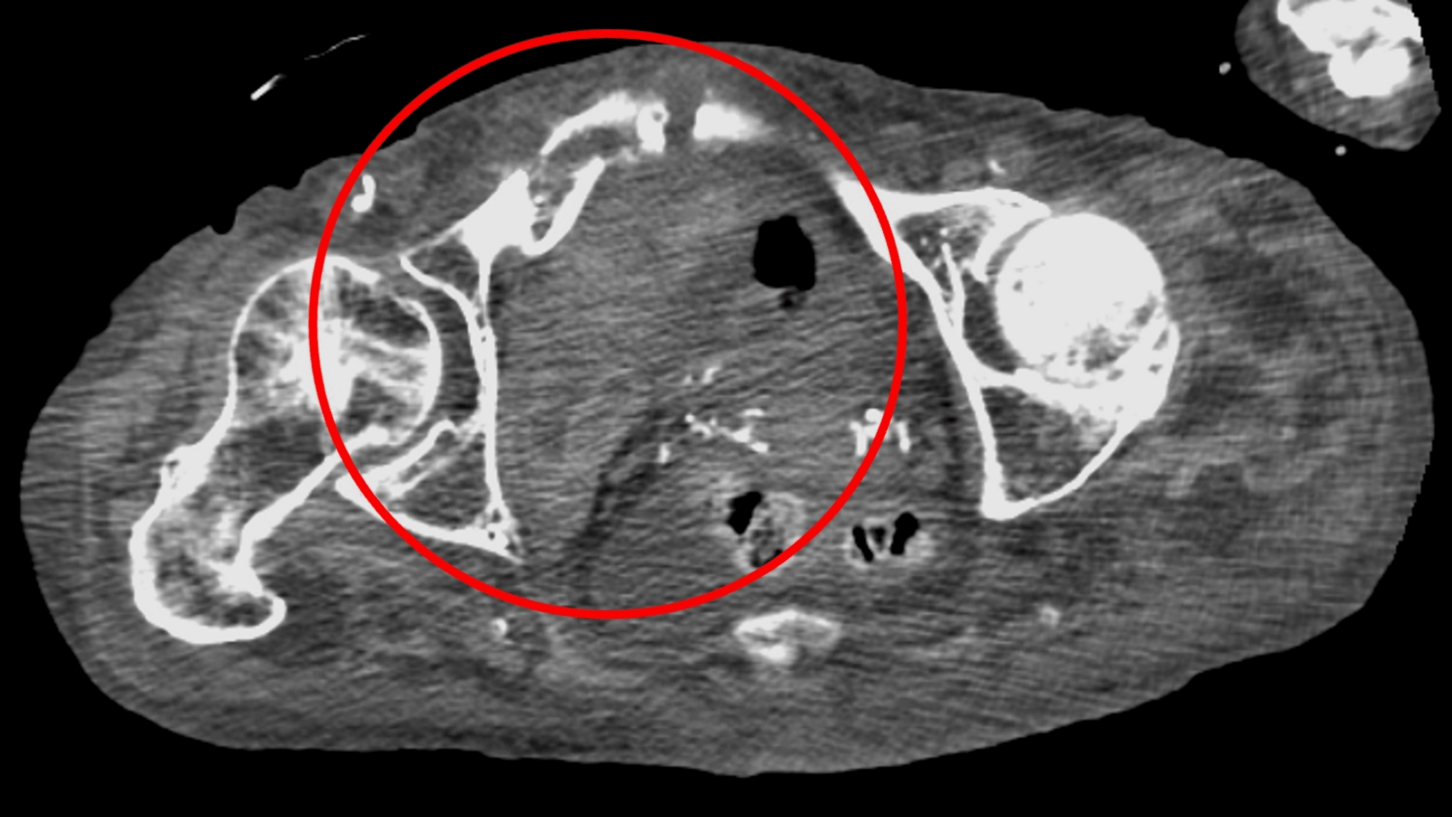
Contrast-enhanced computed tomography showing that the fracture was complicated by injury to the right coronary artery, with a hematoma near the fracture site that gradually enlarged (red circle)
